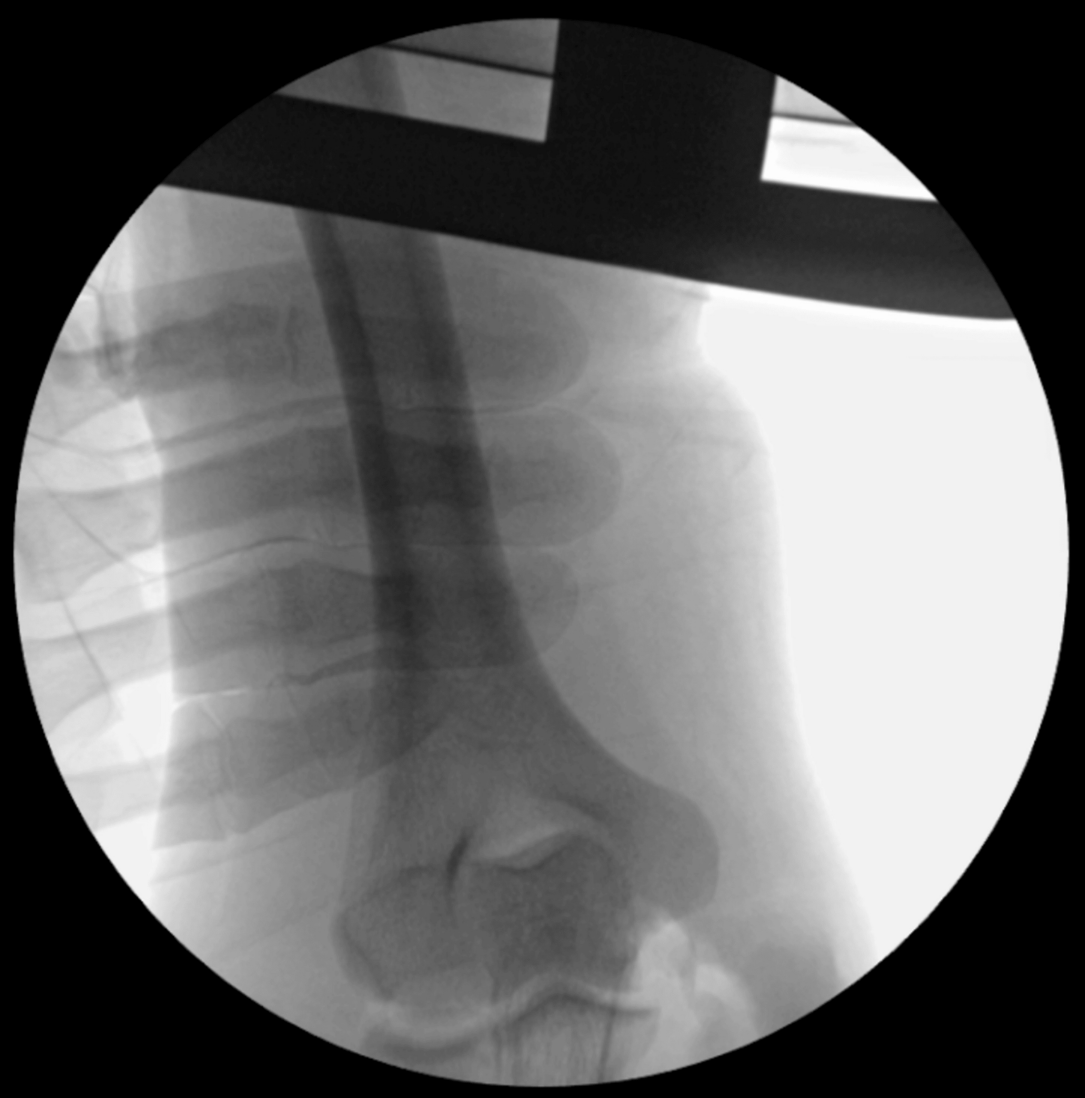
Intraoperative AP X-ray image (fluoroscopy) of the right elbow. AP, anteroposterior
